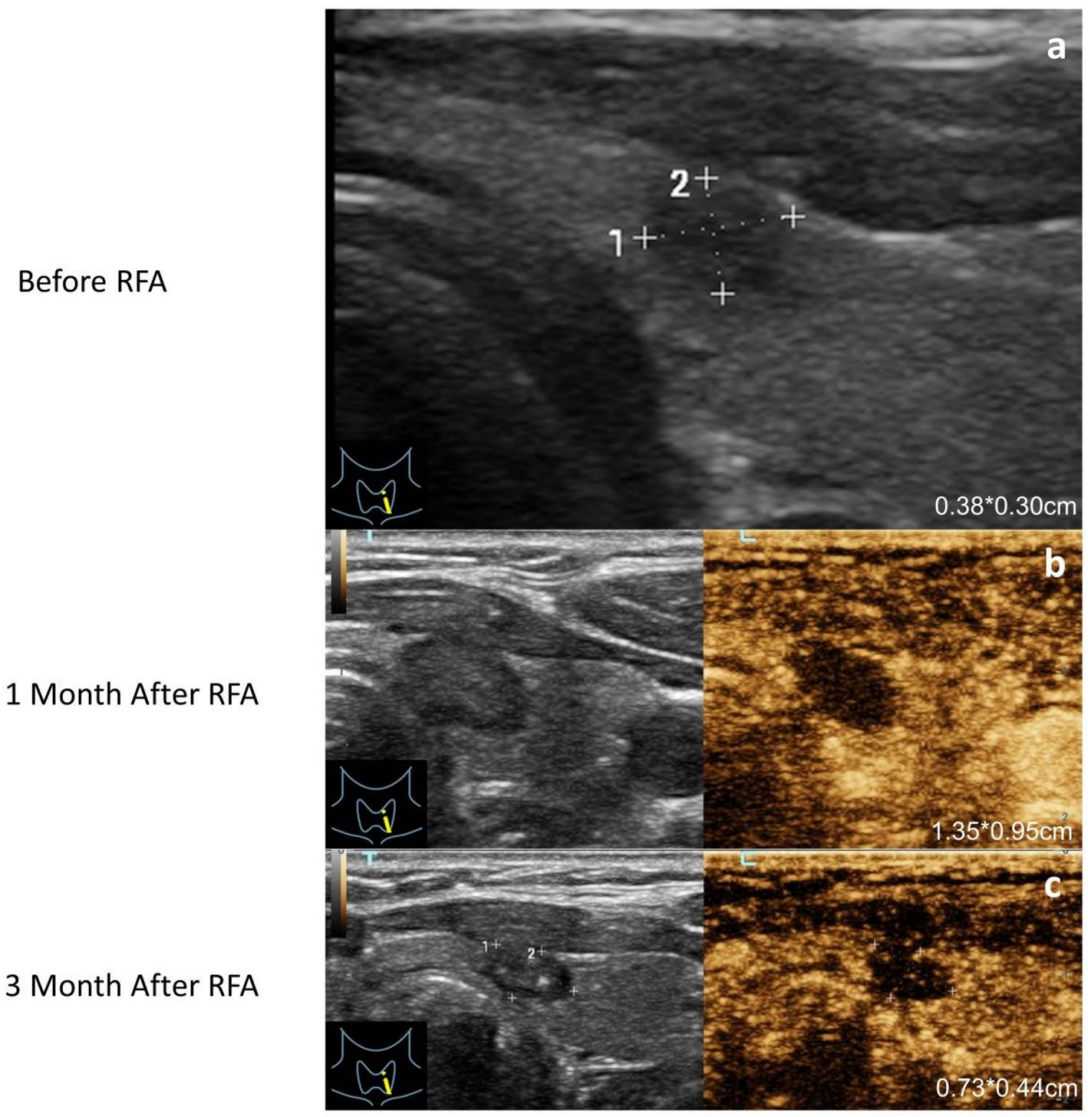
Ultrasound images of PTMC locoregional lymph nodes status in different treatment stages. (a) The original image of PTMC in two-dimensional ultrasound (2D-US). (b) 2D-US and CEUS images of 1 month after PTMC RFA. (c) 2D-US and CEUS images of 3 months after PTMC RFA.
